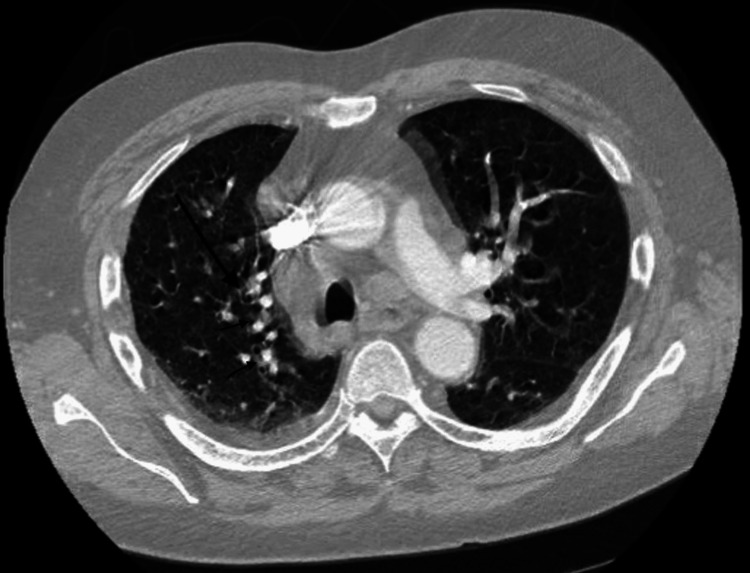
Computed tomography (CT) angiography showing multiple masses within the right perihilar lung and mediastinum, consistent with metastatic disease
